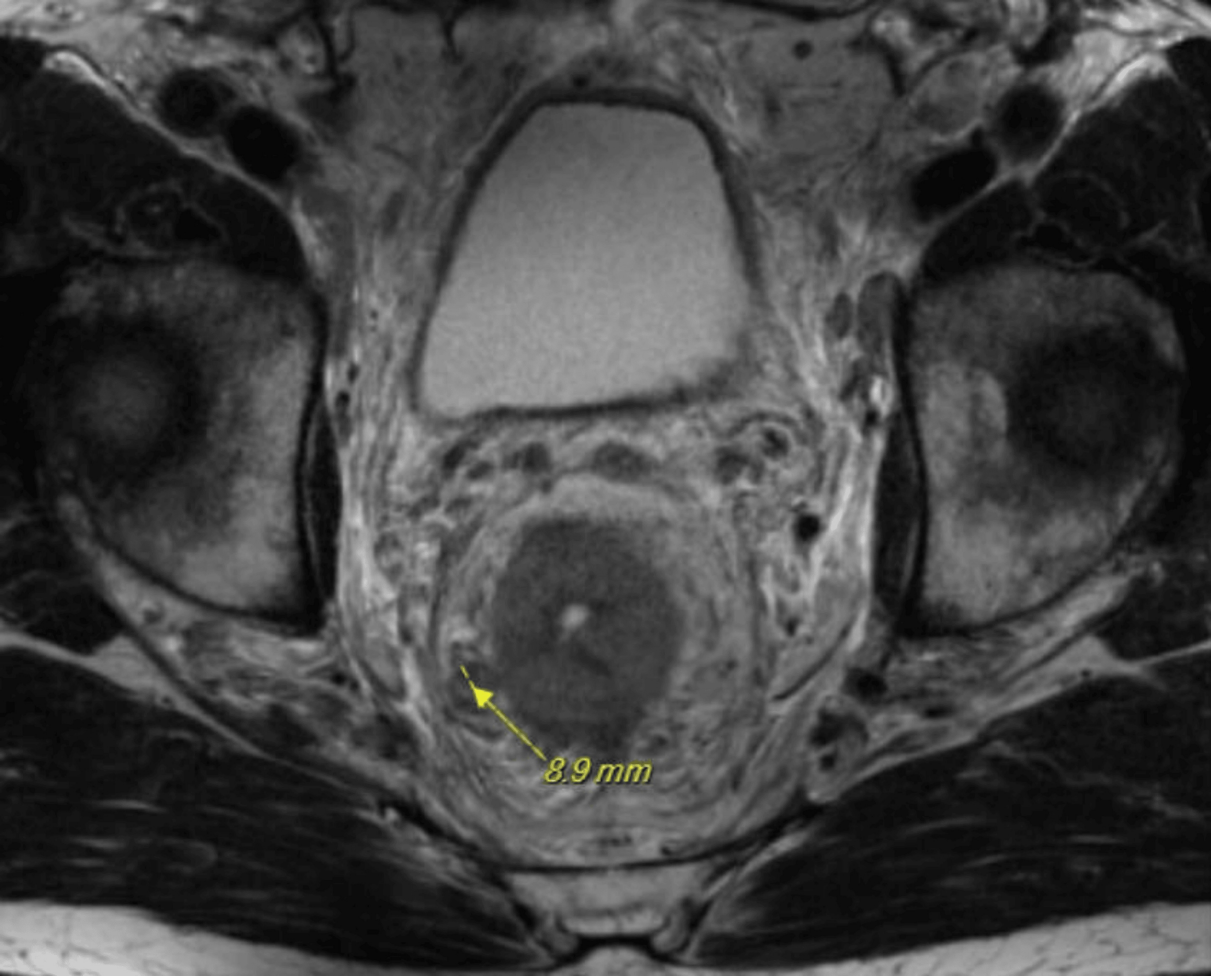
MRI of the pelvis with and without contrast Axial T2-weighted MRI reveals concentric mass-like wall thickening of the rectum and an enlarged mesorectal lymph node measuring 8.9 mm (yellow arrow).
